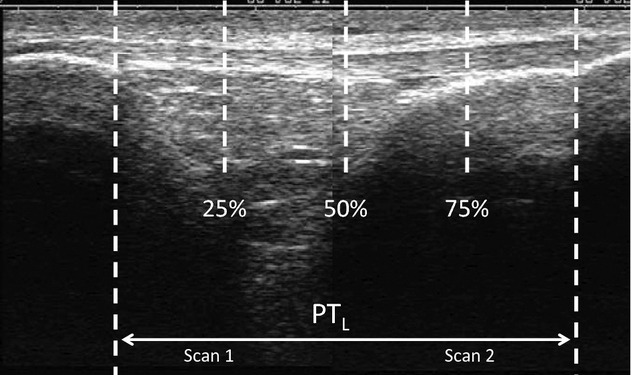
Sagittal plane ultrasound image (composed of two scans) of the patella tendon denoting measurement locations of tendon cross-sectional areas at 25%, 50%, and 75% of total patella tendon length (knee at 90^o^ flexion).
